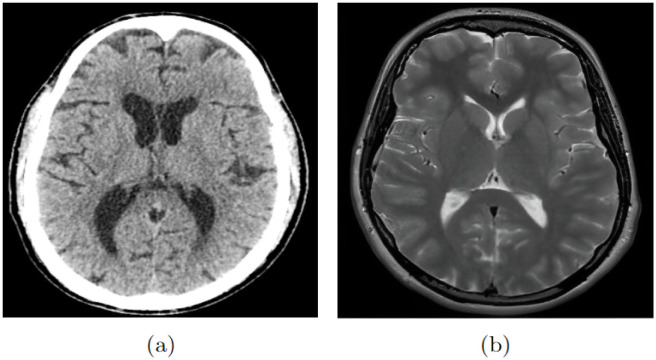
Brain exams: (a) computed tomography e (b) magnetic resonance.
